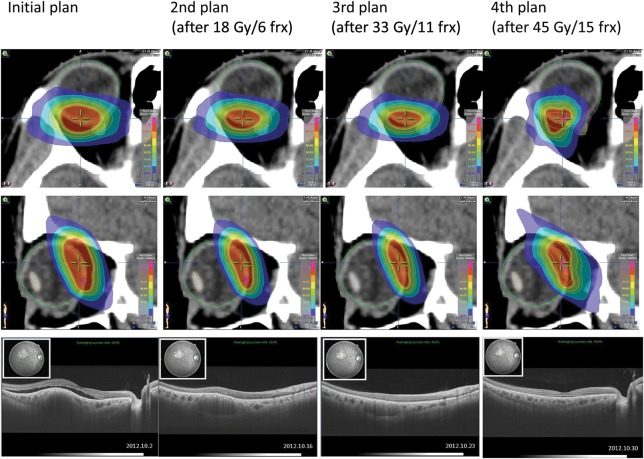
Adaptive replanning IMRT for right choroidal metastasis, based on CT images in axial view (top line) and sagittal view (middle line), in which GTV was defined using information from the SS-OCT image (bottom line). By the fourth plan, the monitor unit was changed to avoid overdoses to the right optic nerve.
